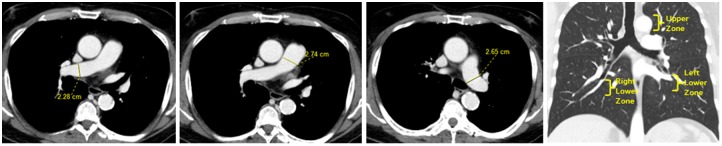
CT scans from a control subject demonstrating bronchovascular measurements. Axial images demonstrate measurements of the main pulmonary artery (A), the right pulmonary artery (B), and the left pulmonary artery (C). Coronal image from the same subject demonstrates location of the upper and lower zones for bronchovascular measurements (brackets) (D).
